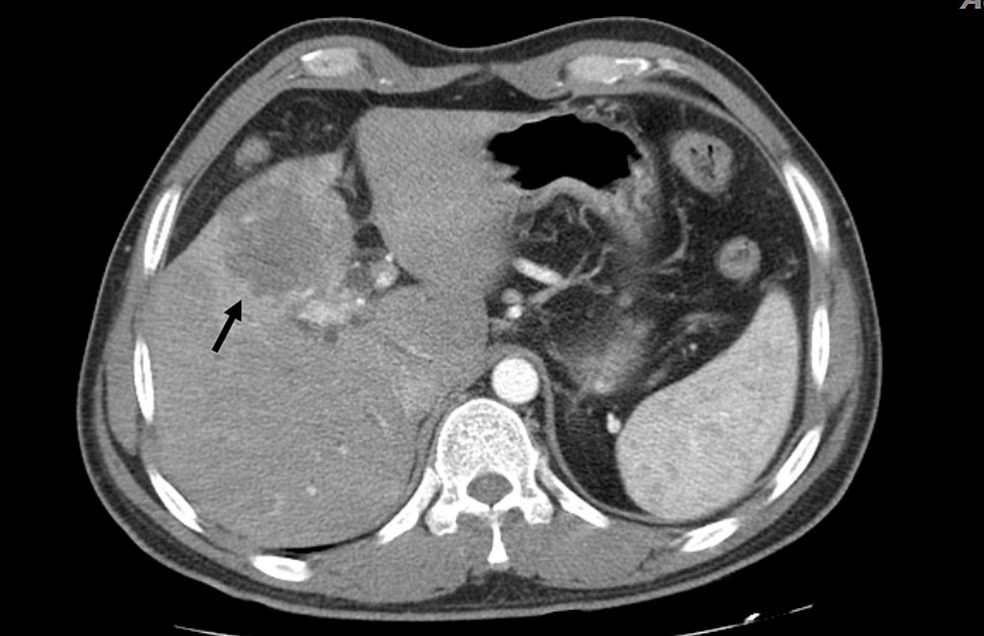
Three-phasic CT scan in the arterial phase of a 45-year-old male, who was a known case of PSC, presented with jaundice. The heterogeneous mass lesion in the medial segment of LT lobe of the liver was associated with capsule retraction and delayed enhancement, indicating cholangiocarcinoma. PSC: primary sclerosing cholangitis; LT: left
